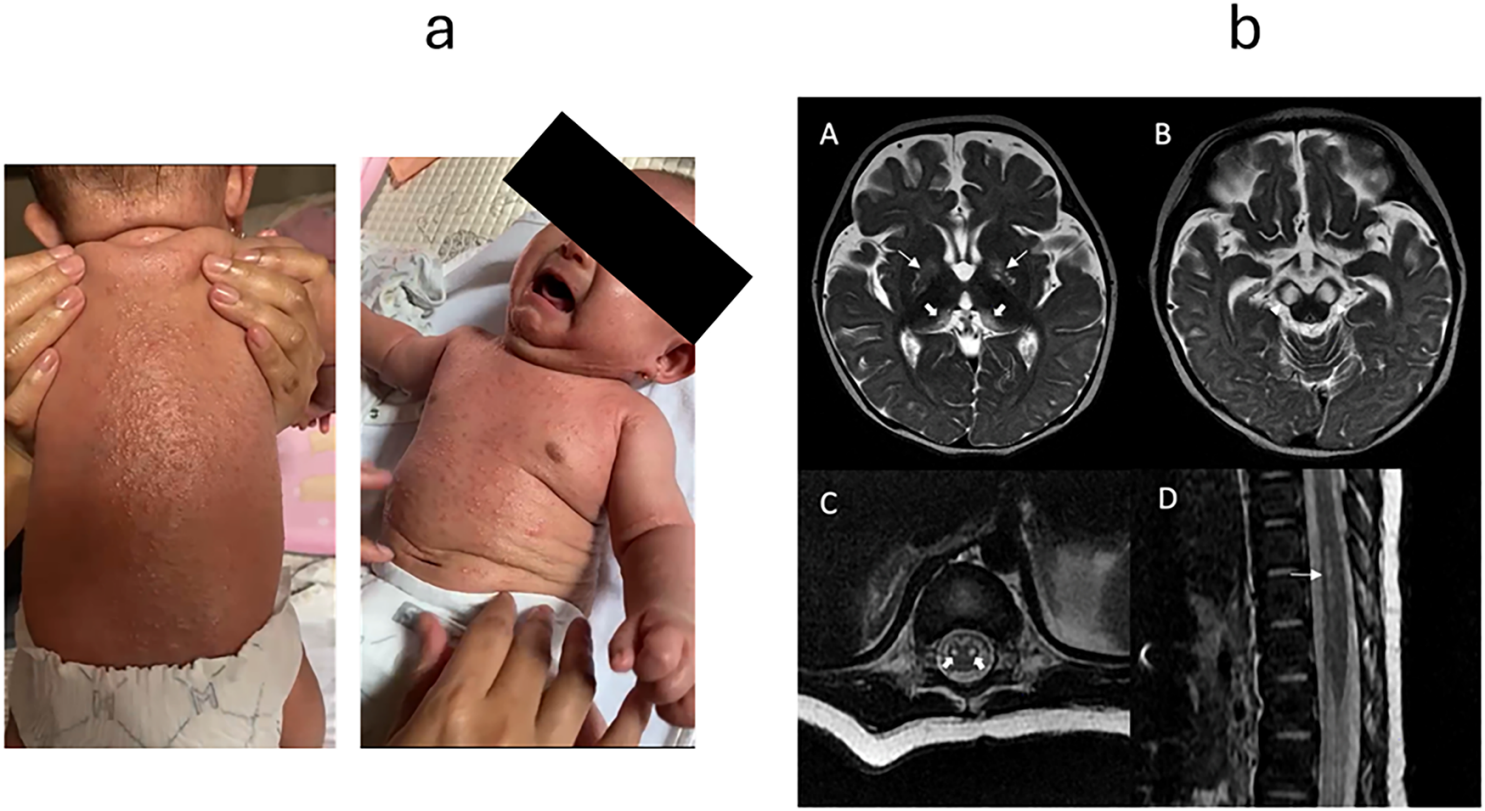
(a) Generalized maculopapular rash before treatment in Indonesia. (b) Magnetic resonance imaging (MRI) brain and spine Selected axial T2-weighted MRI brain images showing symmetrical T2-weighted hyperintense signal involving the (A) globus pallidi (thin arrows), posteromedial thalami (thick arrows), and (B) substantia nigra. Selected axial (C) and sagittal (D) T2-weighted MRI whole spine images showing T2-weighted hyperintense signal involving the anterior horns of spinal cord from T10-T12 level (thick and thin arrows in C and D, respectively).
